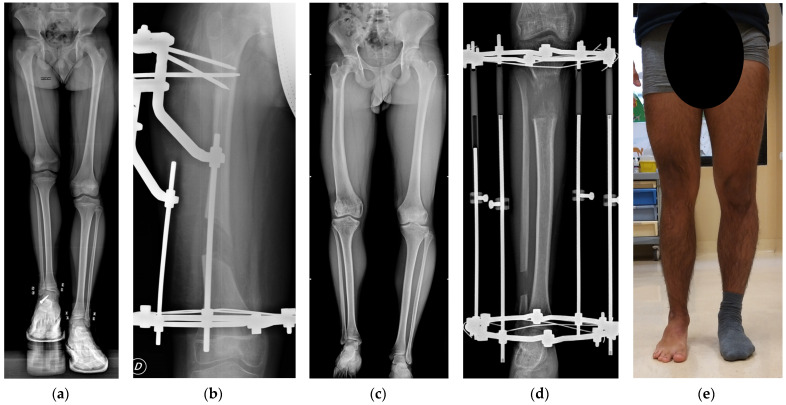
Case of LLD in congenital deficiency of the right femur in a male treated with two lengthening procedures with Ilizarov EFs. (a) Preoperative radiograph at 11 years and 10 months of age (LLD = −9 cm equal to 6% of height). The patient was treated for right flexible flat foot at the age of 9 years with subtalar joint arthroeresis with a non-absorbable talar screw that was removed before the first corticotomy procedure. (b) Radiograph after 50 days of femoral lengthening; after a few days, the patient achieved the planned lengthening of 5.5 cm and the EF was removed 220 days after the corticotomy (HI = 40 days/cm). (c) Pre-operative radiographs at 15 years and 10 months of age (LLD = −7.5 cm equal to 4.5% of height). (d) Radiograph after 60 days of lengthening, in which the patient achieved 6 cm of planned lengthening; patient developed contracture of Achilles tendon; EF was removed 229 days after the corticotomy (HI = 50 days/cm) and Achilles tendon contracture was treated with four-week cast immobilization and subsequently with physical therapy. (e) Clinical picture of lower limbs two years after tibial EF removal. At the last available follow-up, the patient did not complain about pain or limitation during sports, had a full range of motion in the right hip, knee, and ankle, with no signs of articular instability, and had a residual LLD of 1.5 cm, compensated with an insole.
